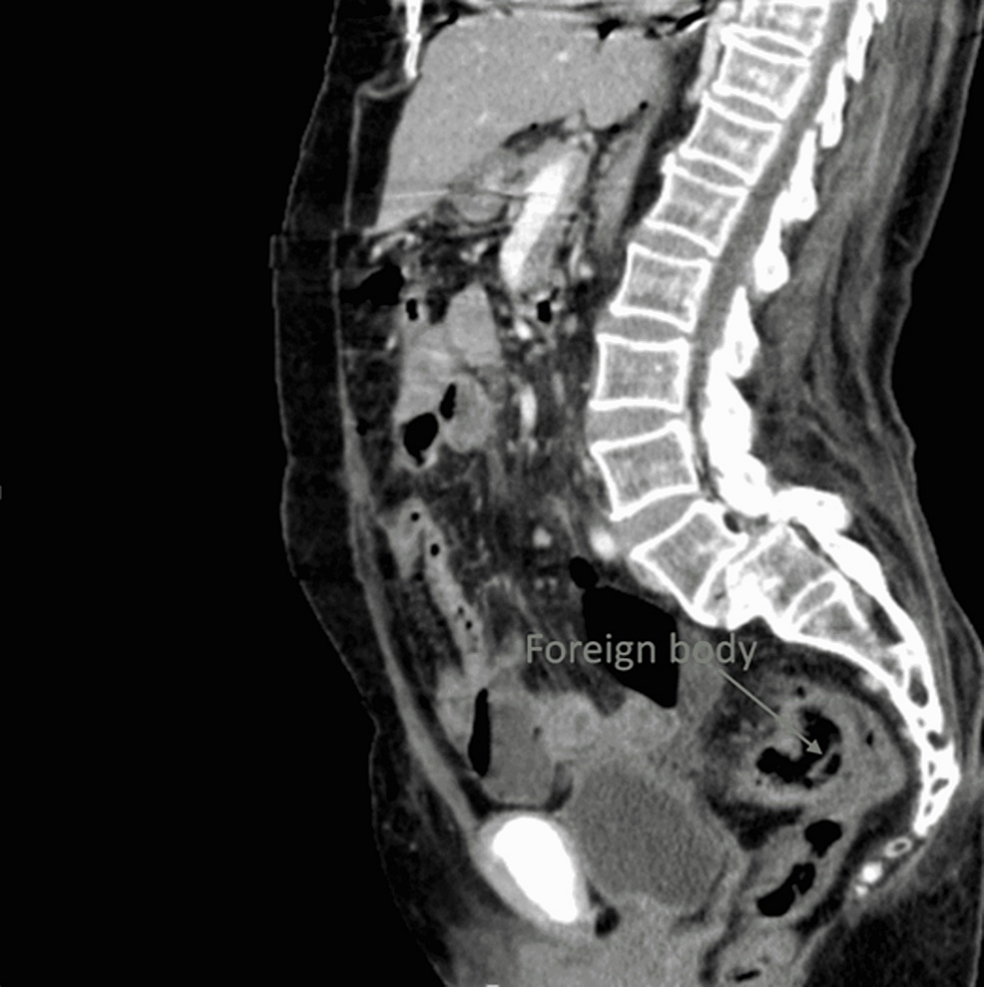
CECT of the abdomen and pelvis (sagittal view) showing visible partial denture at the rectosigmoid junction CECT: High-dose contrast-enhanced computed tomography
